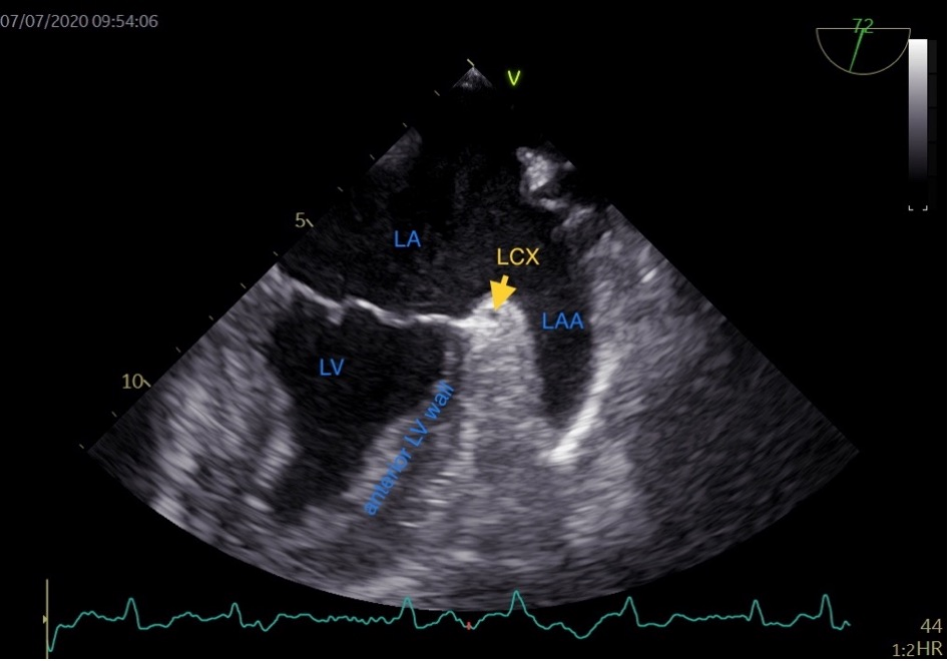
Transesophageal echocardiographic view of the left atrial
